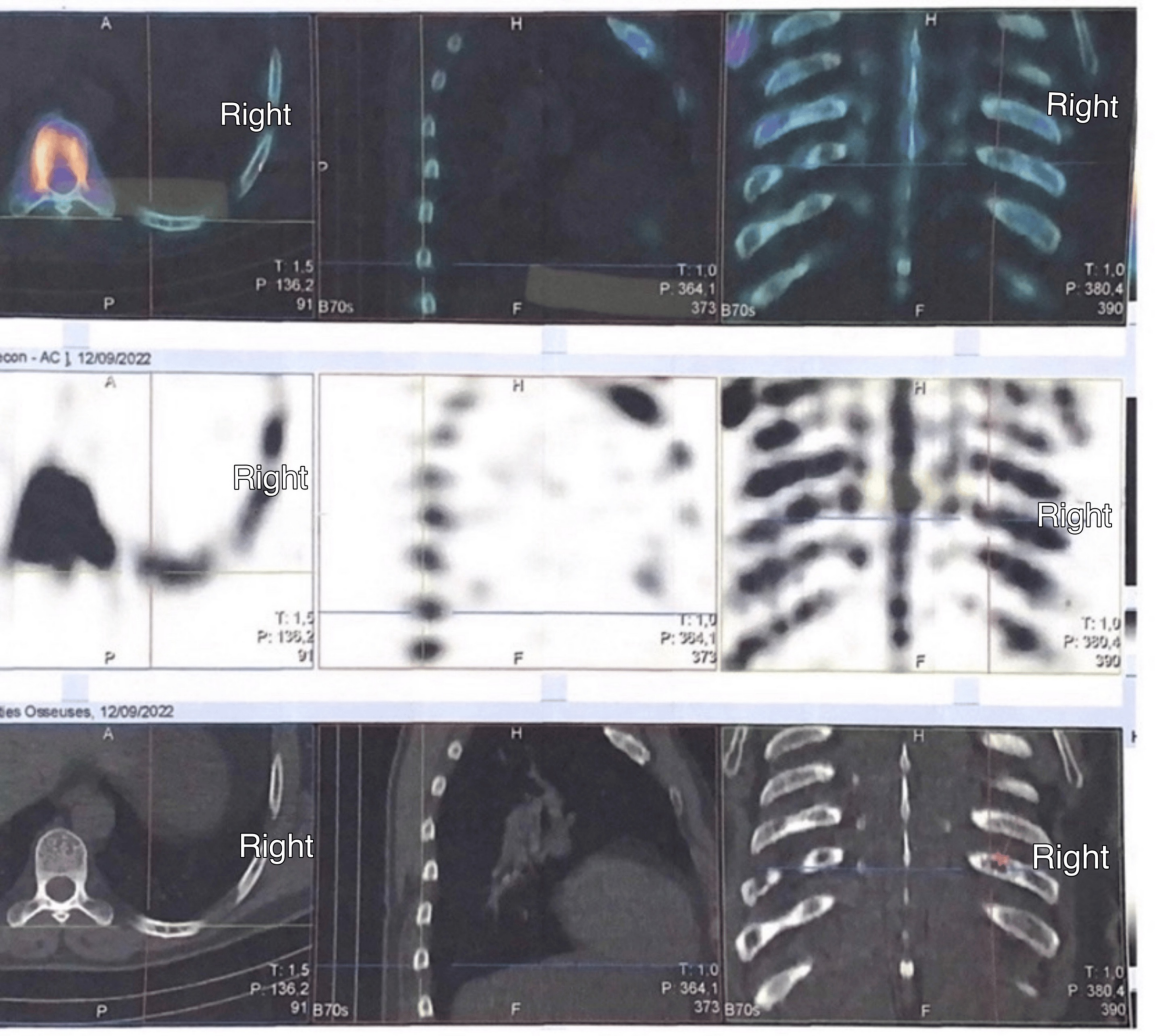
CT findings (axial, coronal, and sagittal views) The images show a sclerotic lesion of the posterior arch of the ninth right rib without cortical breach, suggestive of osteoid osteoma CT: computed tomography
